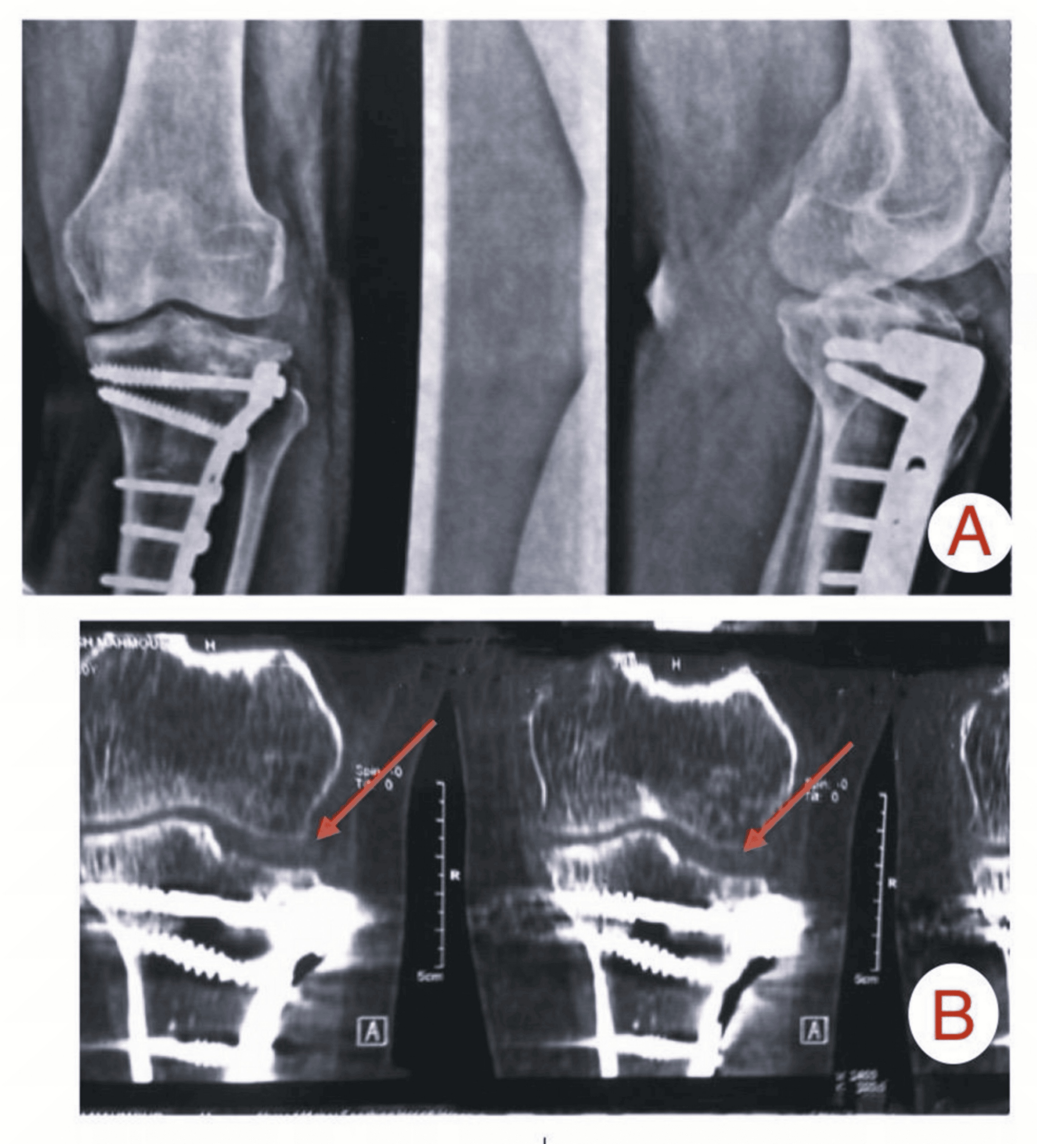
Six-month follow-up (A) X-ray and (B) CT scan images of Patient A showing secondary depression of the reduced lateral tibial plateau articular surface
